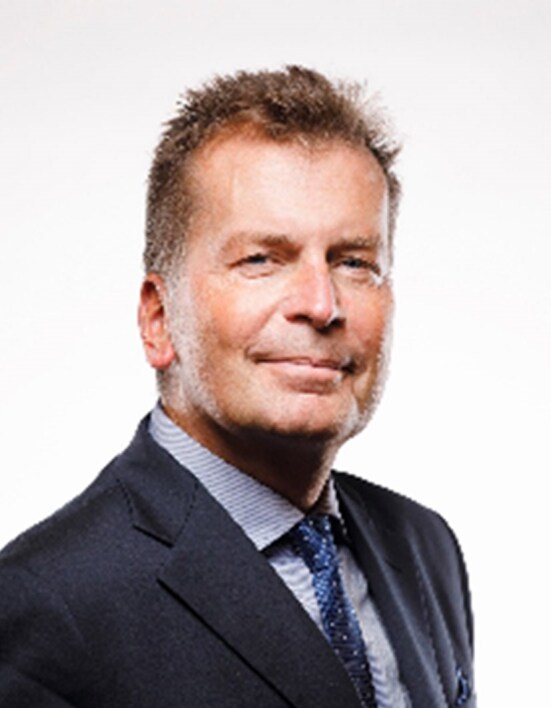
The effect of initiating sacubitril/valsartan (sac/val) therapy during hospitalization for acute heart failure (AHF) on left ventricular (LV) remodelling remains unclear. Dr Tanaka and co-workers from Japan, aimed to assess the impact of Sac/Val on LV remodelling in patients in whom sacubitril/valsartan (Sac/Val) was initiated during AHF hospitalization. The authors used data from Program of Angiotensin–Neprilysin Inhibition in Admitted Patients with Worsening Heart Failure (PREMIER) study, which investigated the impact of initiating Sac/Val during hospitalization for AHF on echocardiographic parameters over an 8-week period, in comparison with the standard renin–angiotensin system inhibitor therapy.^1^
